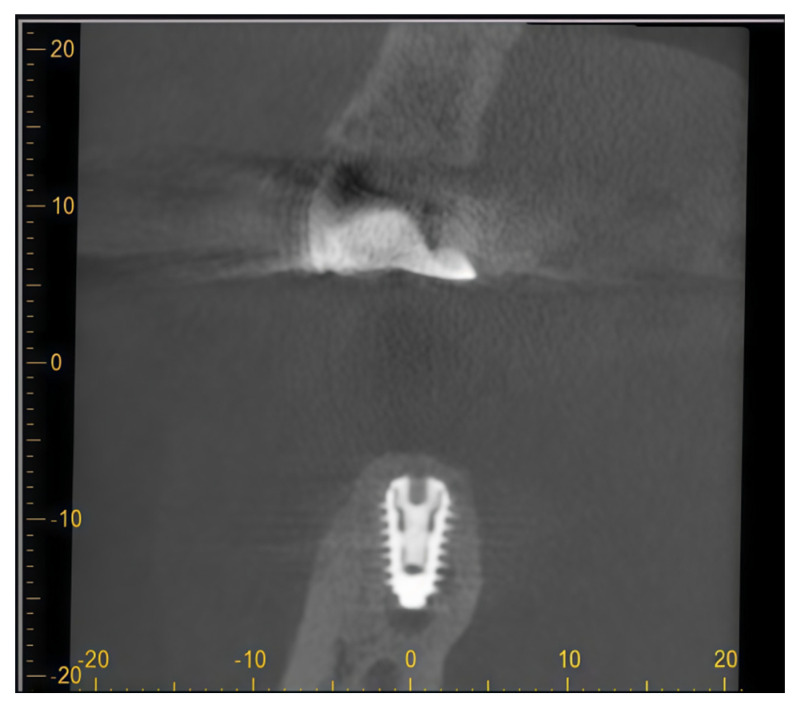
CBCT scan after 6 months of healing.
